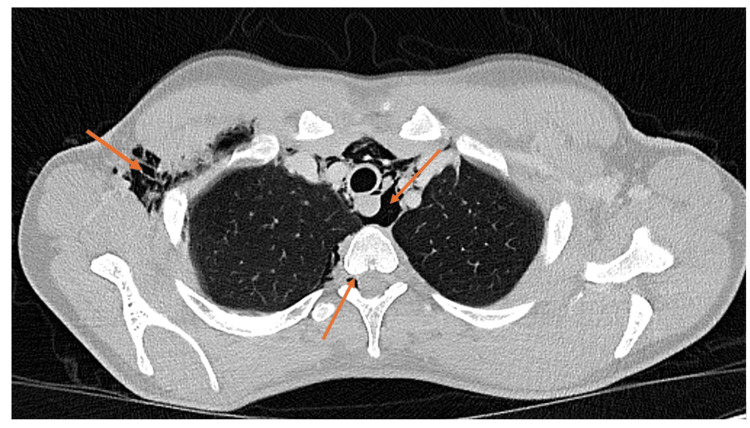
CT scan showing large volume pneumomediastinum with extensive surgical emphysema of the neck and right axilla/chest wall CT: computed tomography
